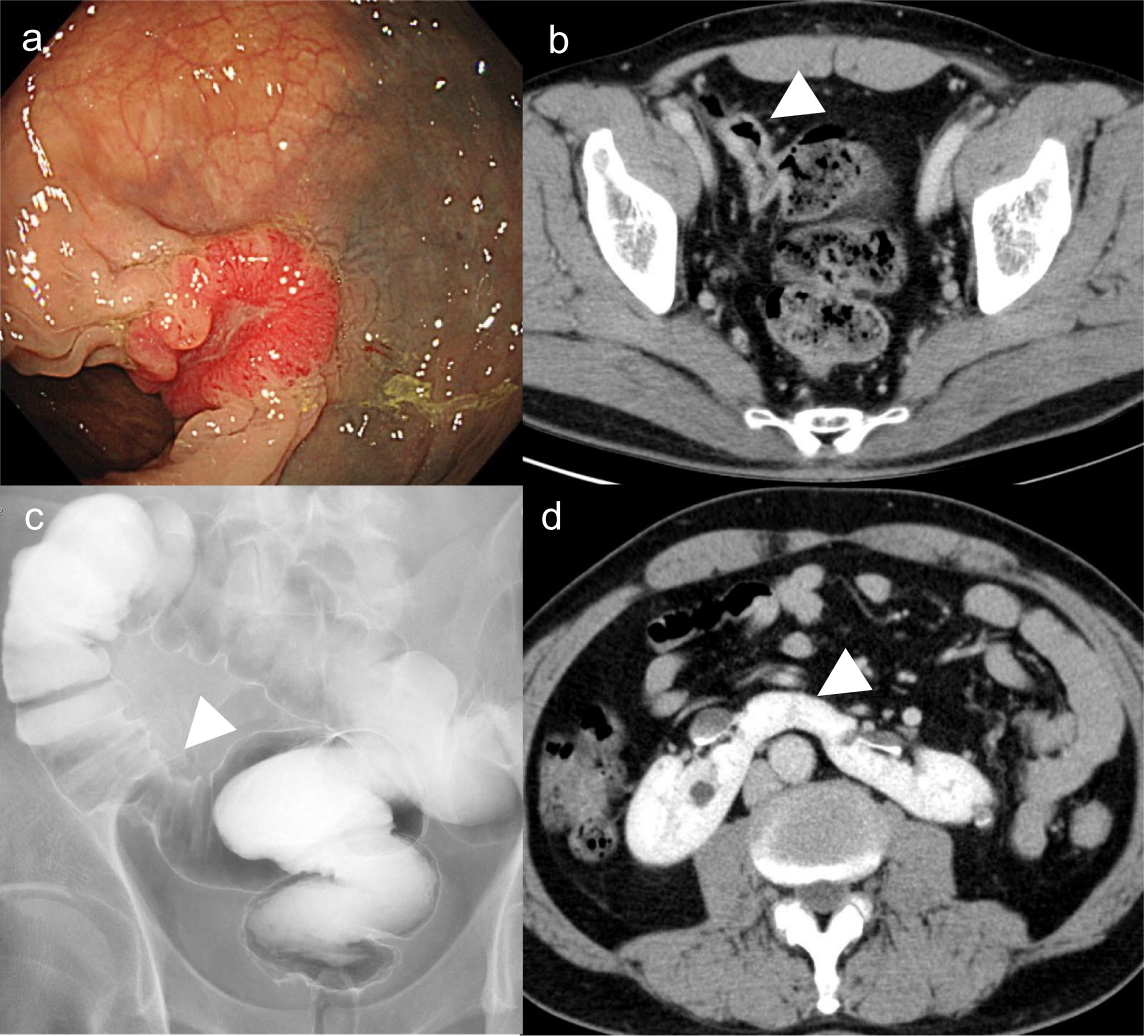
Preoperative findings. a Colonoscopy revealed a type-2 tumor in the sigmoid colon. b A contrast-enhanced computed tomography revealed sigmoid colon cancer (white arrowhead). c A contrast-enema revealed sigmoid colon cancer (white arrowhead). d The isthmus of the horseshoe kidney (white arrowhead)
